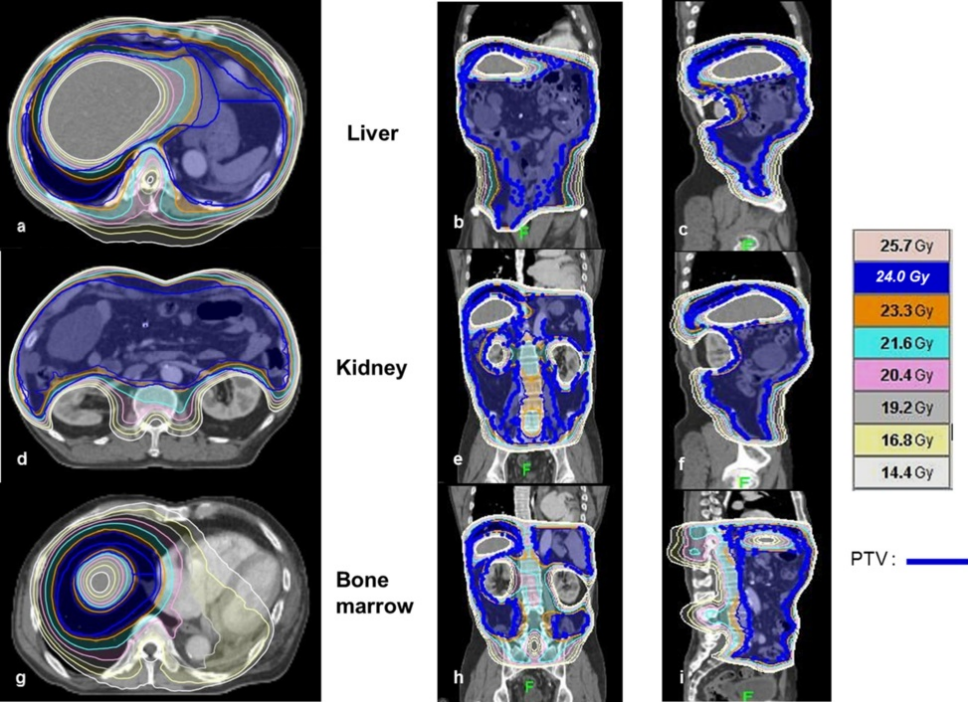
Radiation dose distribution for intraperitoneal radiotherapy using helical tomotherapy-based intensity-modulated radiotherapy, with particular attention to representative dosimetry within the liver (a-c) bilateral kidneys (d-f), and bone marrow (g-i). Transverse (a, d, and g), coronal (b, e, and h), and sagittal computed tomography images (c, f, and i) with isodose radiation color contour maps are provided.
